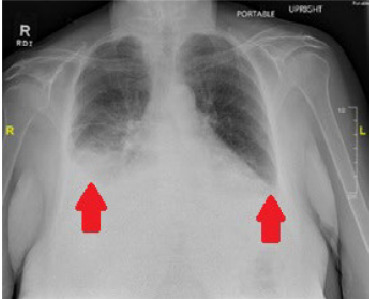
Chest radiograph showing bilateral pleural effusions greater on the right than the left. Arrows point to bilateral pleural effusions.
